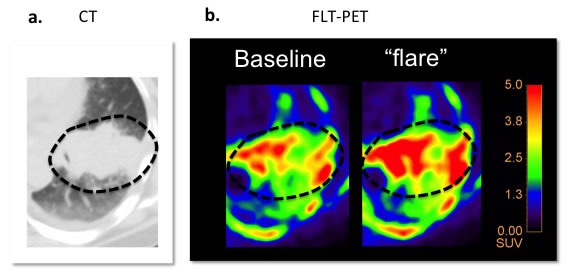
FLT “flare” in response to PEM-based therapy in a human patient These images are from a 63 year-old male with NSCLC participating in our exploratory clinical trial of FLT-PET “flare”. a. Baseline CT revealed a 6 cm mass in the left upper lobe. b. Baseline FLT-PET revealed mild avidity 3 days pre-therapy. c. FLT-PET “flare” imaging performed after 2 hours following administration of combination therapy with PEM and carboplatin revealed a burst in tumor avidity in keeping with the FLT “flare.”
